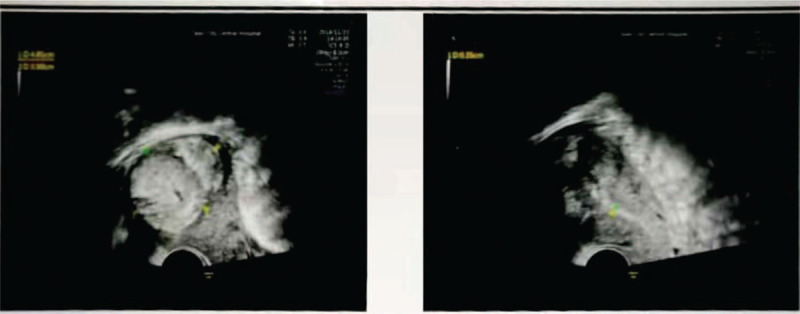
Three months later, ultrasound showed that the endometrial thickness of the middle and lower segment was about 0.35 cm, and that there was a 4.9 × 3.9 cm uneven echo group at the bottom of the uterine cavity, with a strong local echo and no obvious blood flow signal; the outer edge was about 0.24 cm from the thinnest part of the serous layer.
